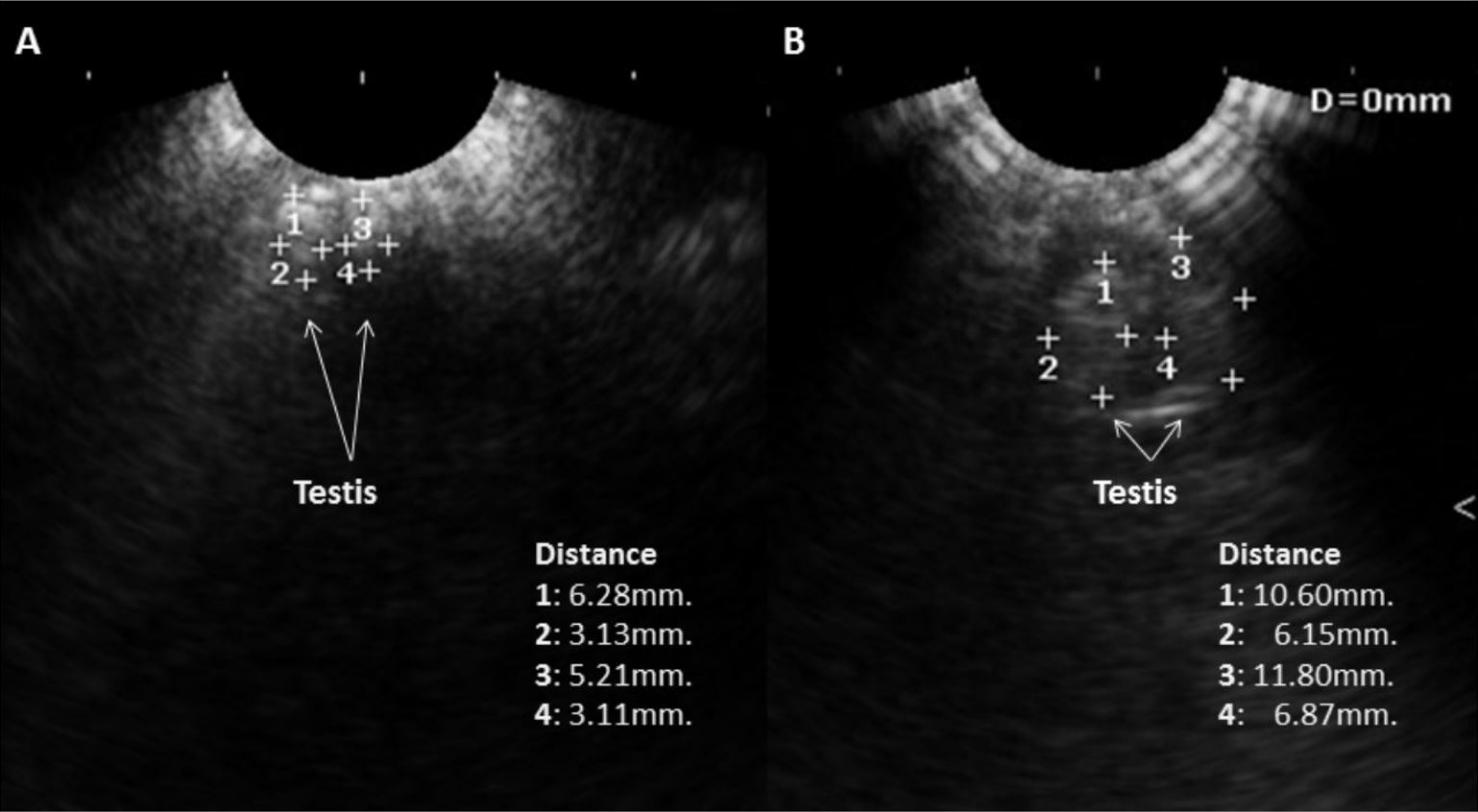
Ultrasonographic image of the testicles of the control group (A) and treated group (B). The (+) associated with 1 and 3 indicate the cranial position of each testicule and the distance to the opposite (+) indicates the length. The (+) associated with 2 and 4 and the distance to the opposite (+) indicates each testicule width.
